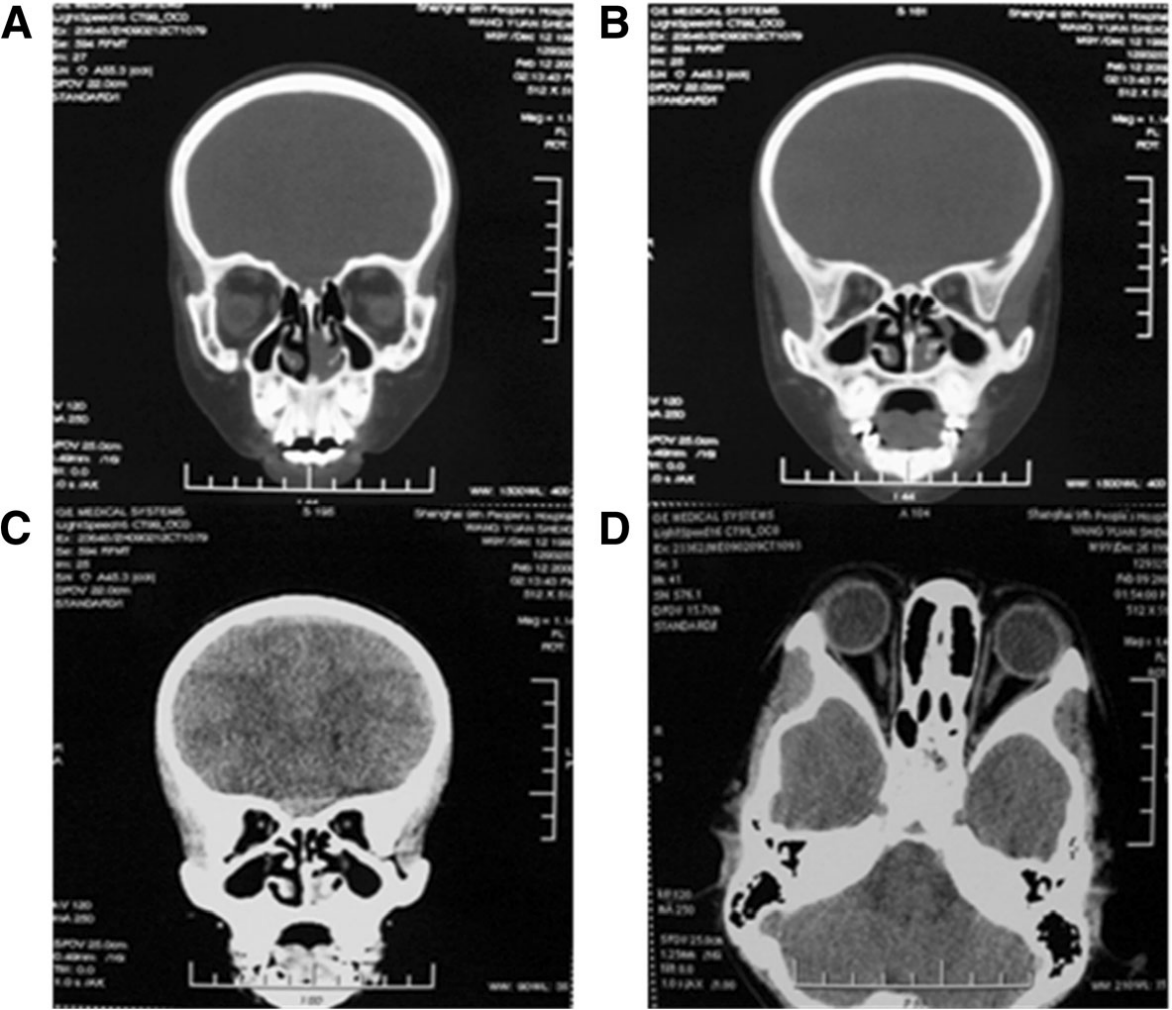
a-d An orbital CT performed in 2009 showed the following: symmetrical line sag in the bilateral anterior maxillary sinuses with normal bone structures in the orbit
